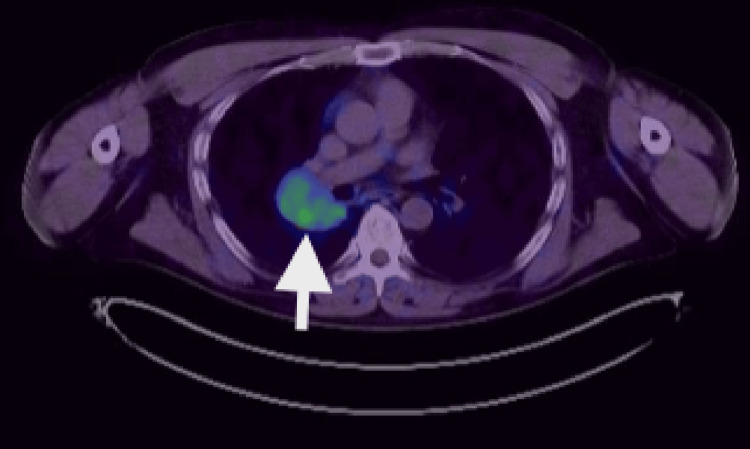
Nuclear medicine Ga-68 DOTATATE PET-CT scan in axial view demonstrating low-grade SSTR uptake (white arrow). Ga-68: gallium-68, PET: positron emission tomography, SSTR: somatostatin receptor.
